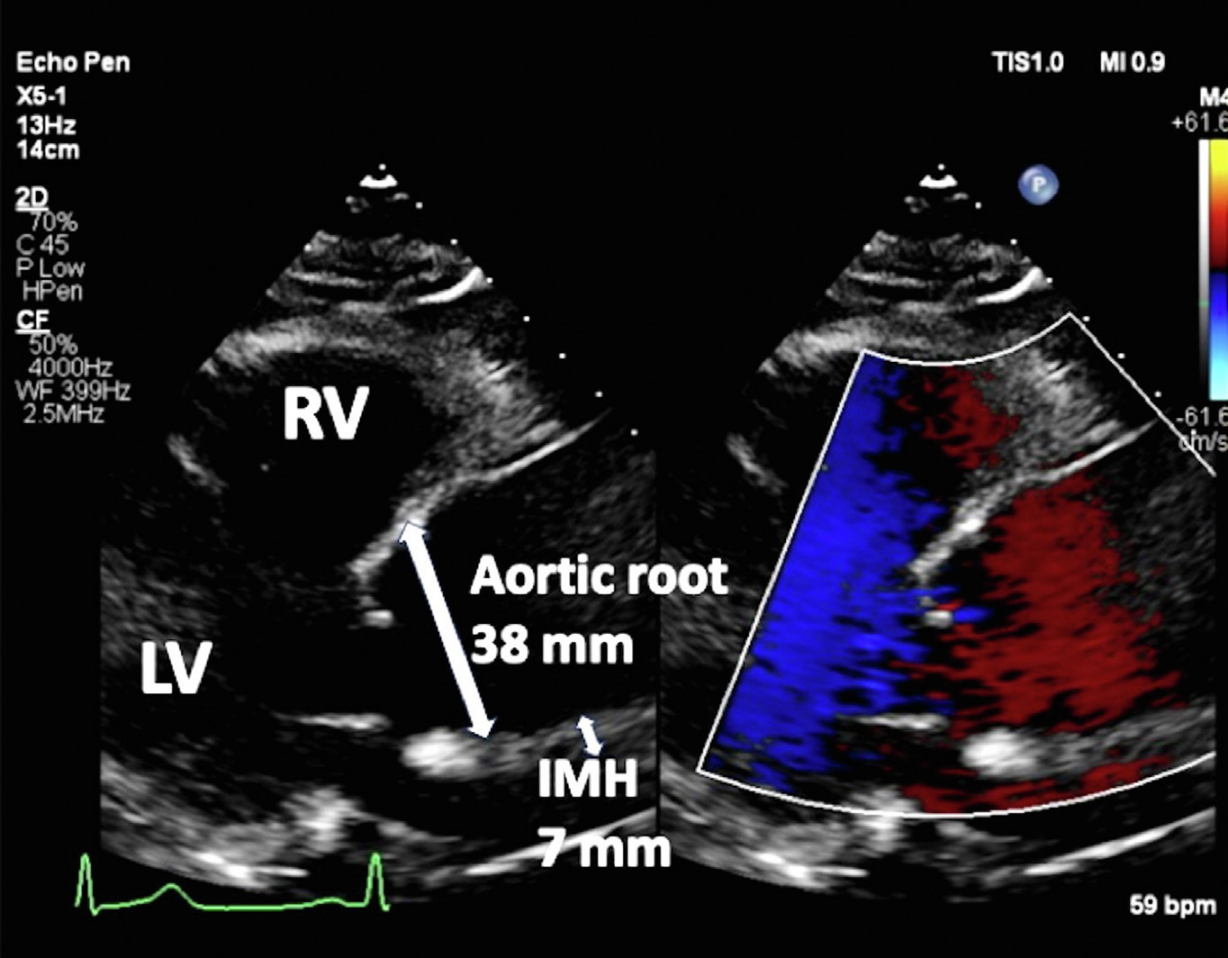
Two-dimensional TTE, parasternal long-axis view without (*left*) and with (*right*) color-flow Doppler in diastole, demonstrates a normal sized, 38 mm aortic root (*long arrow*) with abnormal aortic wall thickening consistent with the 7 mm IMH (*small arrow*; this was only noted on retrospective review of these images). There is no false lumen or aortic dissection flap seen. *RV*, Right ventricle.
